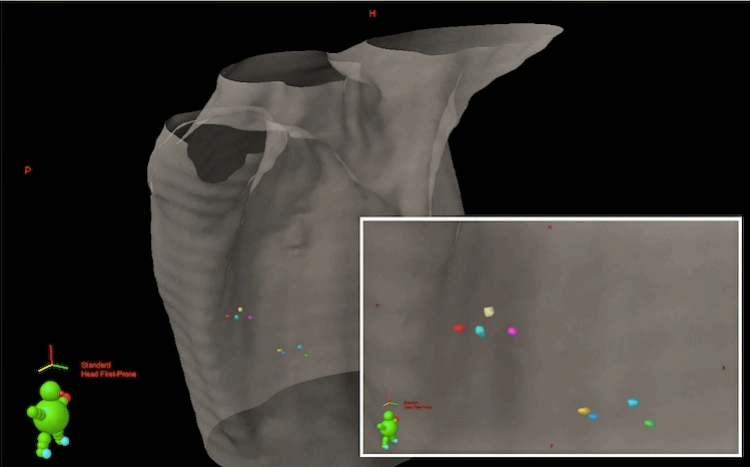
CT scan with markers contoured (with inset). Markers were implanted around two separate right lung tumors.
